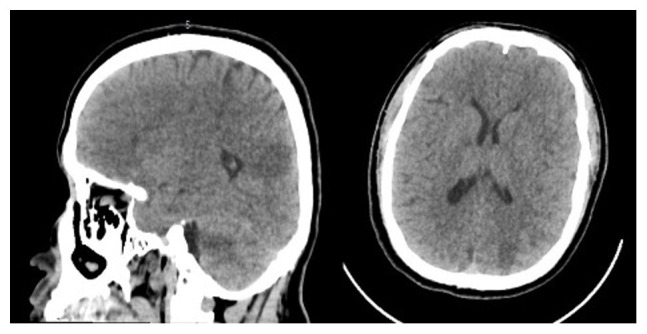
contrast-enhanced computed tomography (CT) scan showed embolic cerebral infraction consisting in multiple supratentorial regions of hypodensity in a vascular distribution occurring in the white matter-gray-matter.
